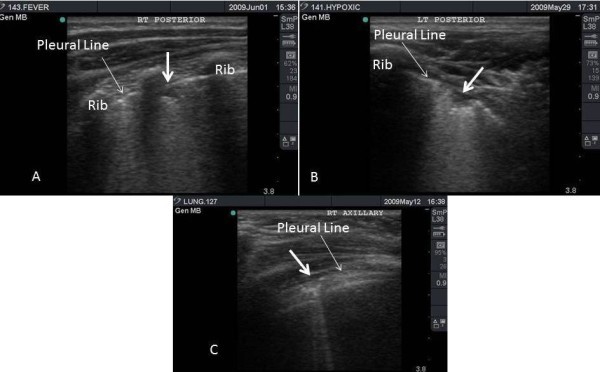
Small subpleural consolidations (arrows) with trailing comet tail artifacts consistent with viral pneumonia lung ultrasound pattern. (A, B, and C) are images of small subpleural lung consolidations in three different patients with suspected H1N1.
